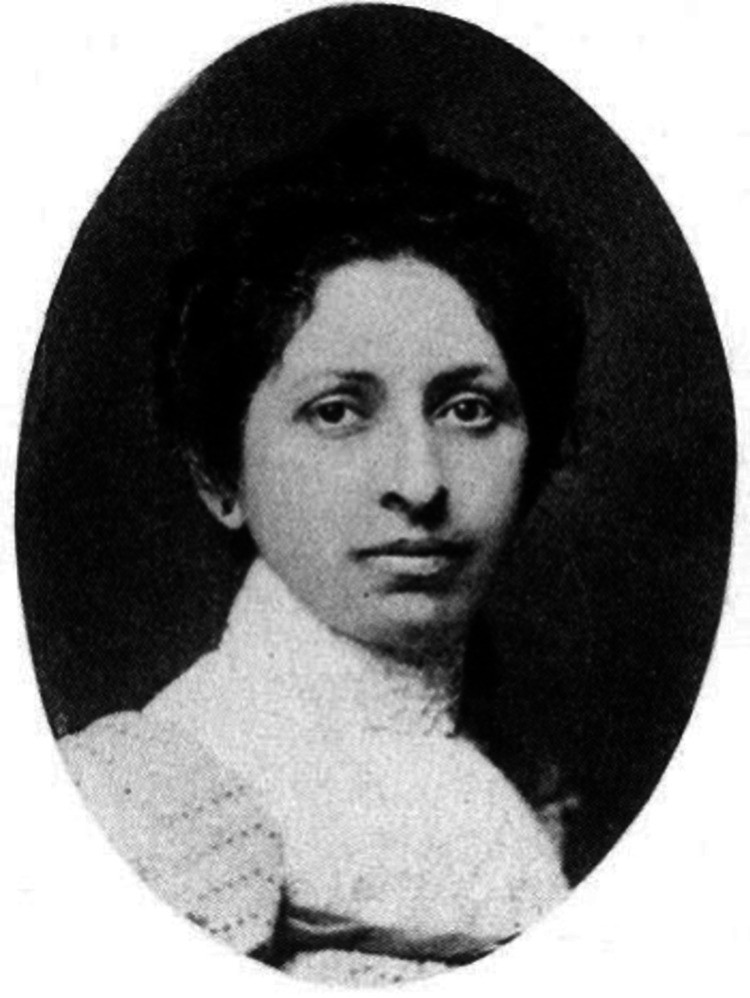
Portrait of Elizabeth Fleischmann-Aschheim From: https://www.foundsf.org/index.php?title=X-ray_Photographer:_Elizabeth_Fleischmann
